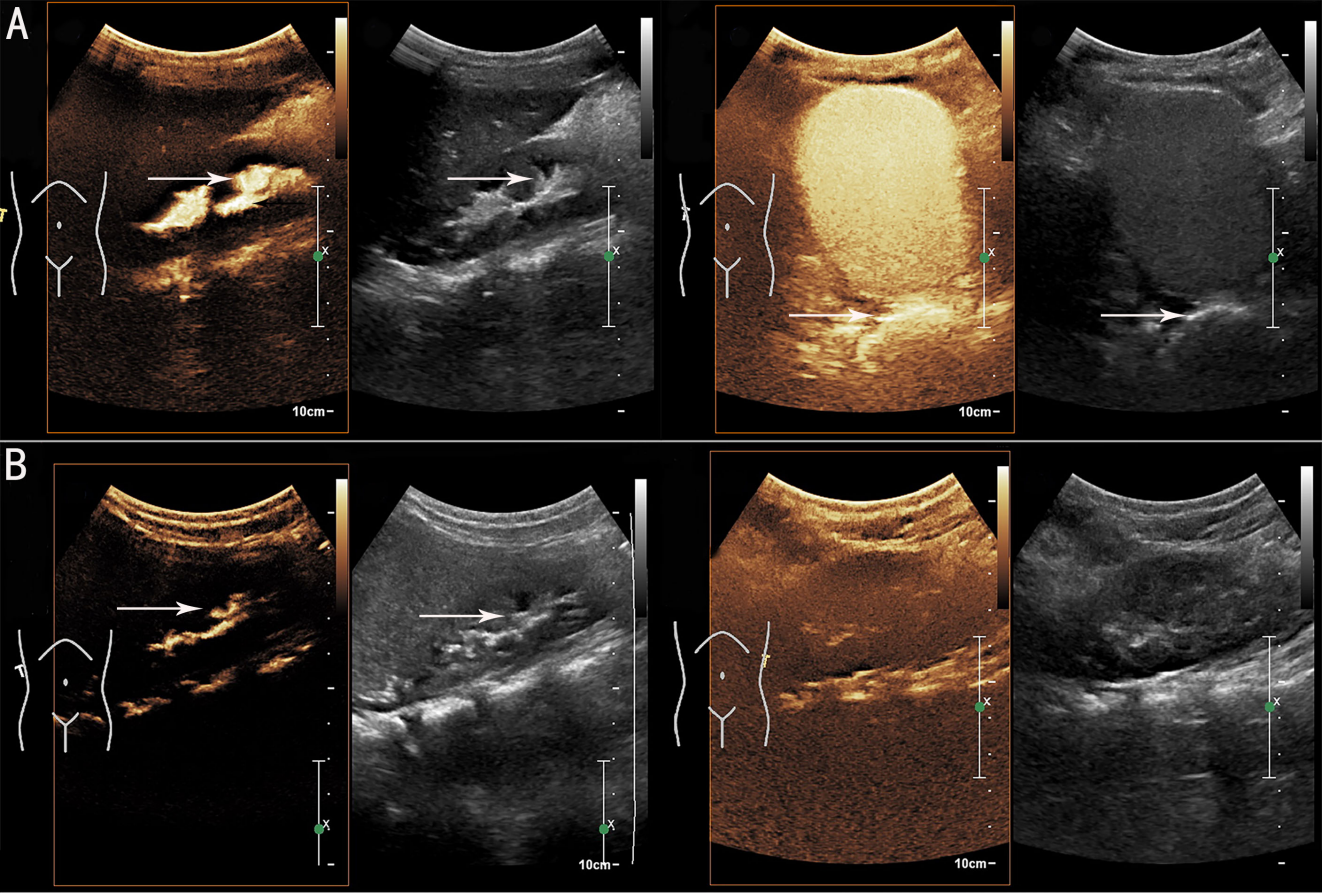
Postoperative contrast-enhanced voiding urosonography (ceVUS) results. (A) ceVUS showed bilateral vesicoureteral reflux (VUR) (grade III on right side and grade I on left side) (white arrows) 4 weeks postoperatively. (B) ceVUS revealed grade II VUR on right side (white arrows) and no VUR on left side 8 months after surgery.
